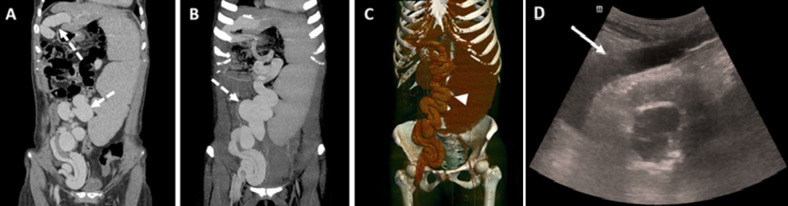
recanalization of abdominal wall veins in portal hypertension: A,B) coronal computed tomography; C) 3D reconstruction, and ultrasound; D) ultrasound showing the patency and flow within the umbilical vein with prominent umbilical and inferior epigastric veins (dotted arrows), measuring 23mm and 31mm respectively, consistent with Cruveilhier-Baumgarten syndrome
